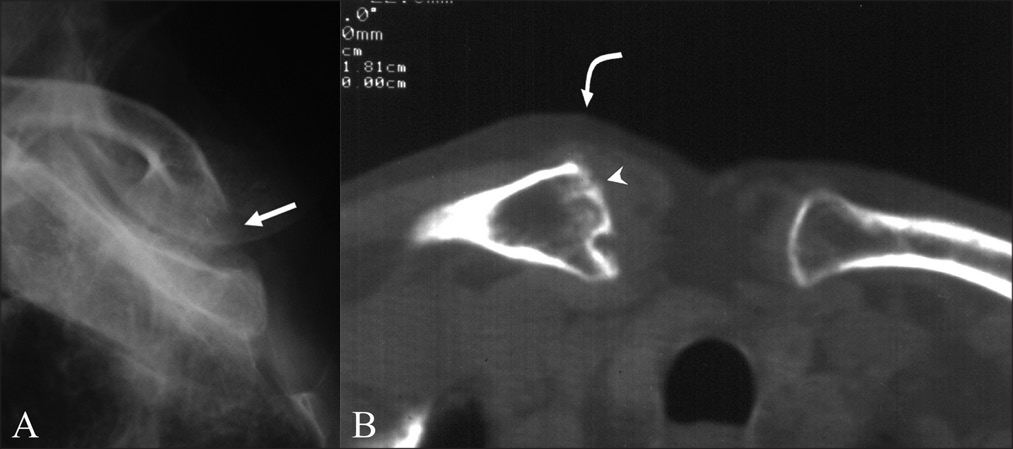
Sterno clavicular tuberculosis – patient 4. Oblique radiograph of the sternoclavicular joint (A) shows an osteolytic lesion (arrow) on the medial articular surface of the right clavicle. CT scan of the sternoclavicular joint (B) shows multiple erosions and expansion of the medial end of the right clavicle (arrow head). Adjacent soft tissue thickening is seen (curved arrow)
